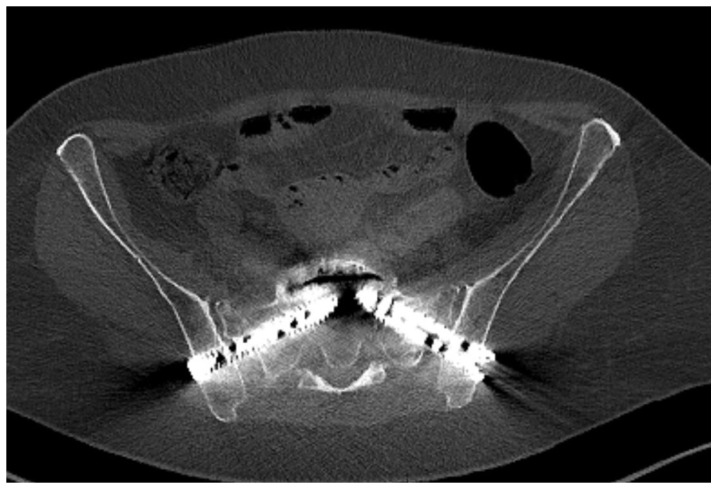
Postoperative axial CT verification of the modified S1 trajectory. The image demonstrates bilateral threaded cylindrical titanium fusion implants (13 mm diameter, 75 mm length) following the planned modified S1 corridor with complete intraosseous containment. The trajectory bypasses the sacral ala region and is directed toward the cranial-ventral S1 body, without evident cortical breach or foraminal encroachment.
